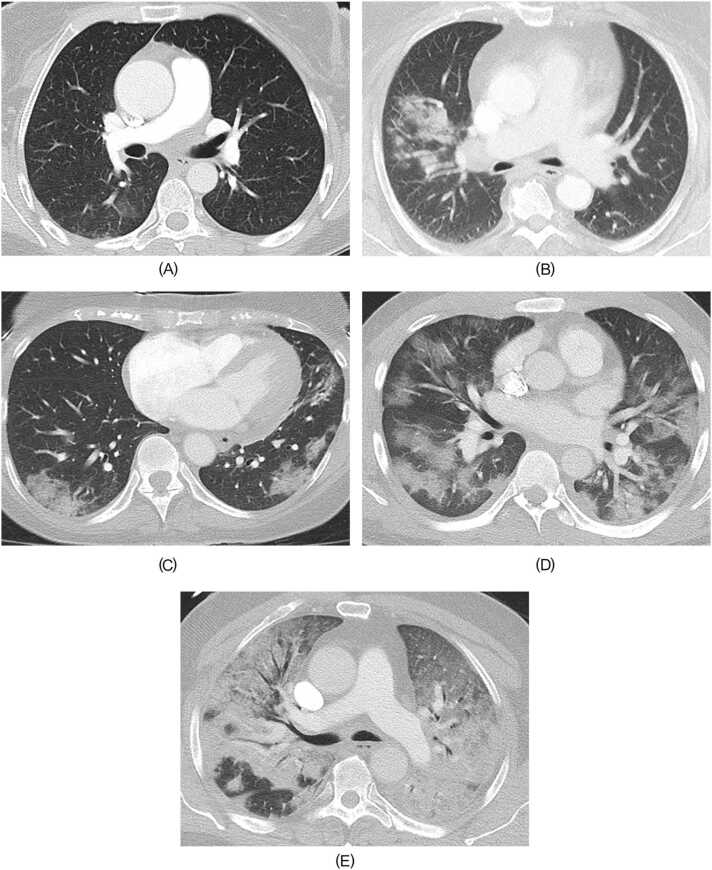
Representative cases for Pneumonia Score 1–5 A. Pneumonia Score 1. 39-year-old woman with hypertension, who had booster or additional vaccination. The time from last vaccination to COVID-19 diagnosis was 4.7 months. Initial CT was performed five days after diagnosis. Subtle GGOs were confirmed in right lower lobe. B. Pneumonia Score 2. 55-year-old woman with asthma, who had complete vaccination series. The time from last vaccination to COVID-19 diagnosis was 6 months. Initial CT scan was performed one day after diagnosis. Focal GGOs and parenchymal consolidation were confirmed in right upper lobe. C. Pneumonia Score 3. 62-year-old woman with a history of lung cancer treatment, who had complete vaccination series. The time from last vaccination to COVID-19 diagnosis was 4.7 months. CT performed eight days after diagnosis showed multifocal bilateral parenchymal consolidation and GGOs, which is consistent with non-extensive OP pattern. Follow- up CT about 2 months later showed that the consolidation and GGOs have mostly resolved. D. Pneumonia Score 4. 29-year-old man without any comorbidities, who had no vaccination. CT performed on the same day of COVID-19 diagnosis showed multifocal and extensive GGOs or consolidations accompanying focal spared areas, which is consistent with extensive OP pattern. E. Pneumonia Score 5. 69-year-old man with hypertension, who had no vaccination. CT performed on the same day of COVID-19 diagnosis showed diffuse and extensive consolidations and GGOs predominantly in dependent lung region accompanying focal spared areas in right lower lobe, which is consistent with DAD pattern. GGOs, ground-glass opacities; OP, organizing pneumonia; DAD, diffuse alveolar damage.
